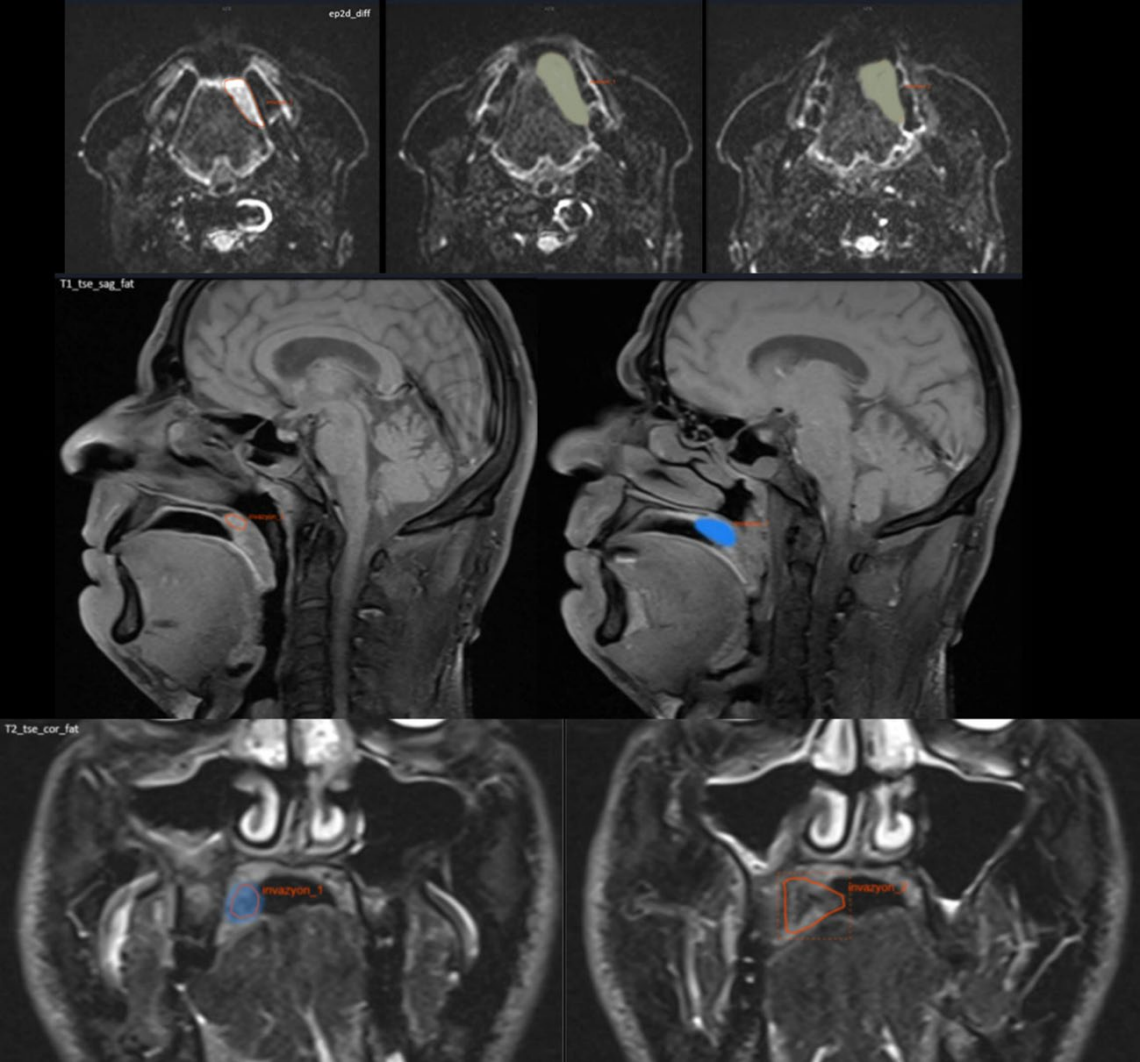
MR showing the segmentation of bone invasion using SVM ML model with manual segmentation and AI segmentation on axial, sagittal and coronal MR images with different sequences
